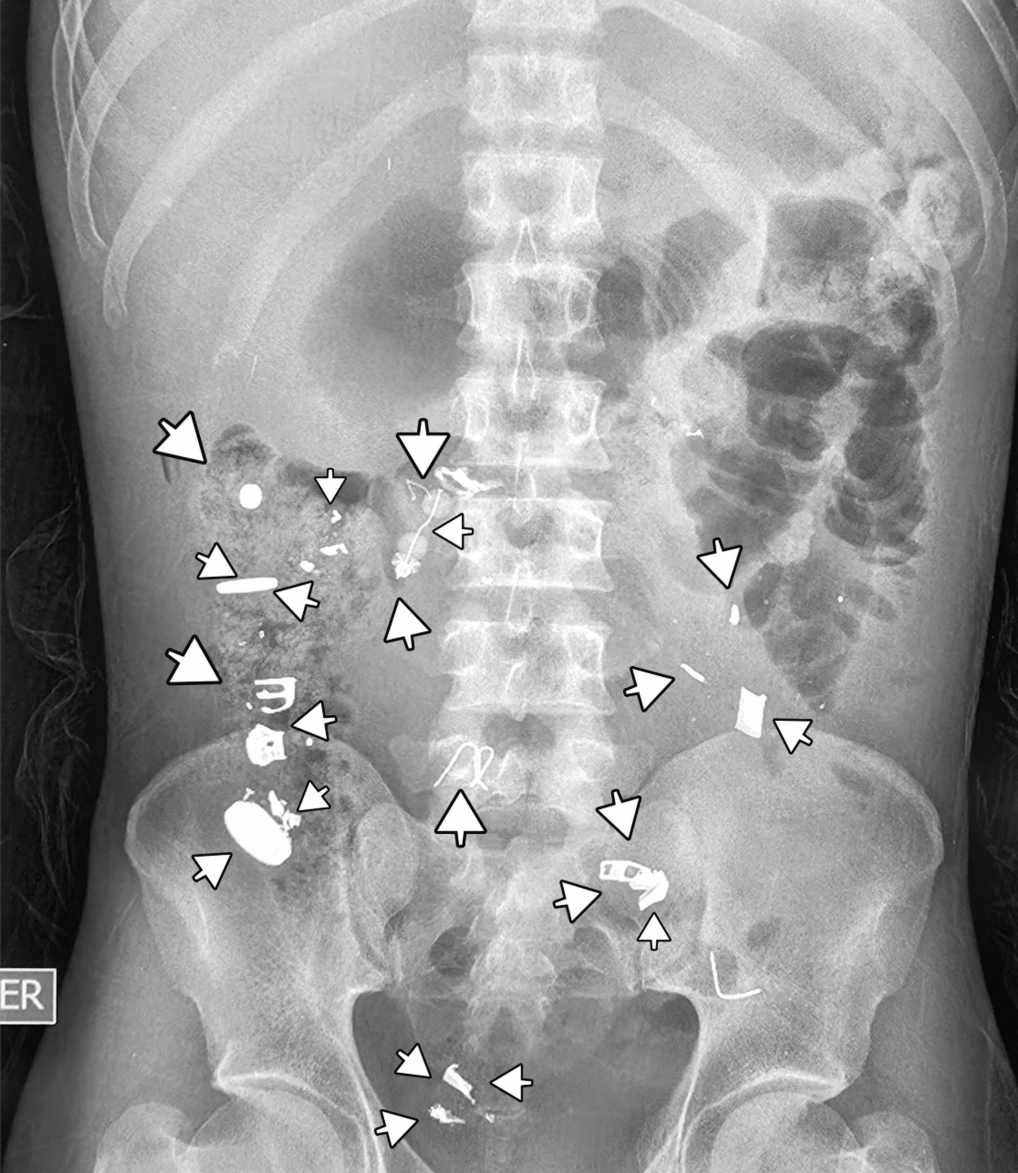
Plain abdominal X-ray showing metallic foreign bodies (arrows) in the colon.
